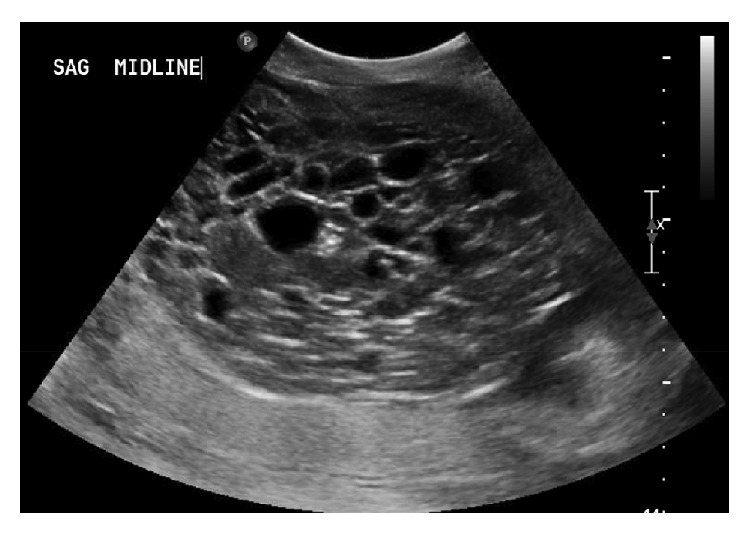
Ultrasound appearance of the molar component of the pregnancy.
